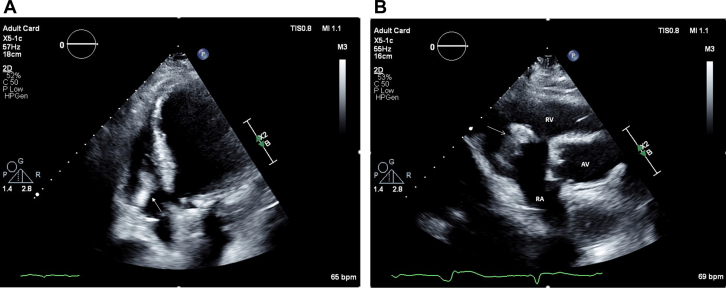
Transthoracic Echocardiography of Echodense Mass (A) Apical 4-chamber view revealing a 4-cm echodensity involving the tricuspid valve near the atrial pacing lead and attached to posterior tricuspid leaflet (arrow); this echodensity was found to be prolapsing back and forth across the valve, with mild tricuspid regurgitation. (B) Parasternal short-axis view showing echodense mass attached to posterior tricuspid valve (arrow). AV = aortic valve; RA = right atrium; RV = right ventricle.
